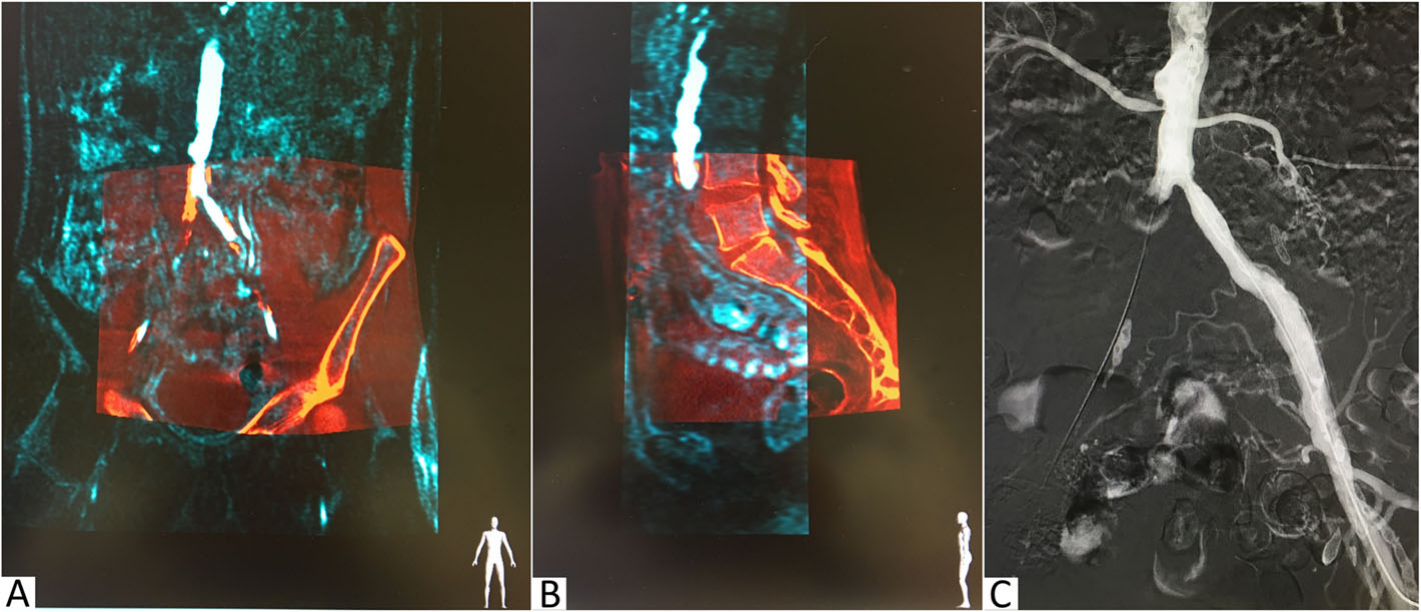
Example of fluoroscopy with MRA fusion image guidance. a, b Coronal and sagittal view of the image registration process (the cone-beam CT images in *red* and the MRA images in *blue*). c An example of the fusion image guidance during a digital subtraction angiography
